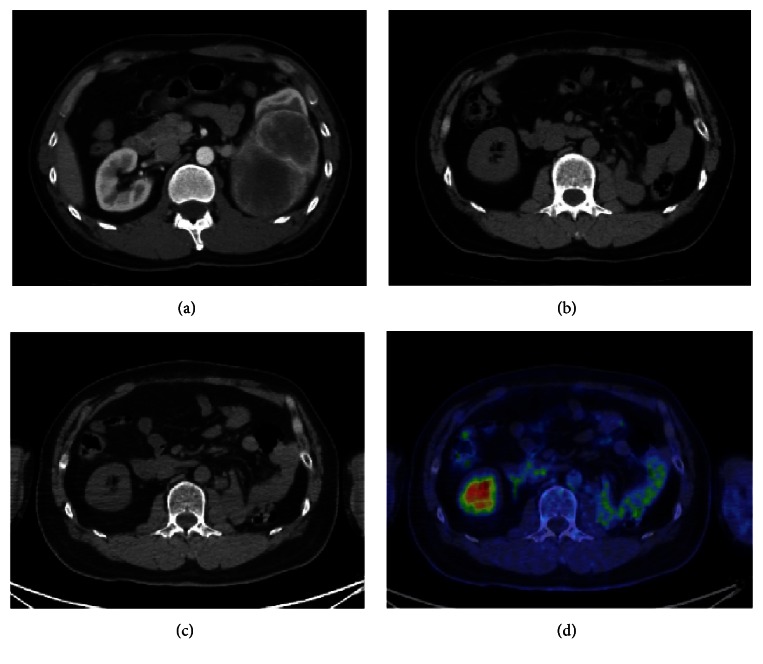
Radiological findings of the renal SFT. Enhanced abdominal CT revealed 17 × 11 × 8 cm tumor located in the left kidney (a). Follow-up plain CT revealed suspicions of recurrent tumor (1 × 0.7 cm) in the tumor bed at 3 years after the nephrectomy (b). Three months after the CT, which detected suspicions of recurrent tumor, follow-up CT scan and PET-CT were performed. (c, d) The mass lesion was increased in size (1.7 × 1.1 cm).
